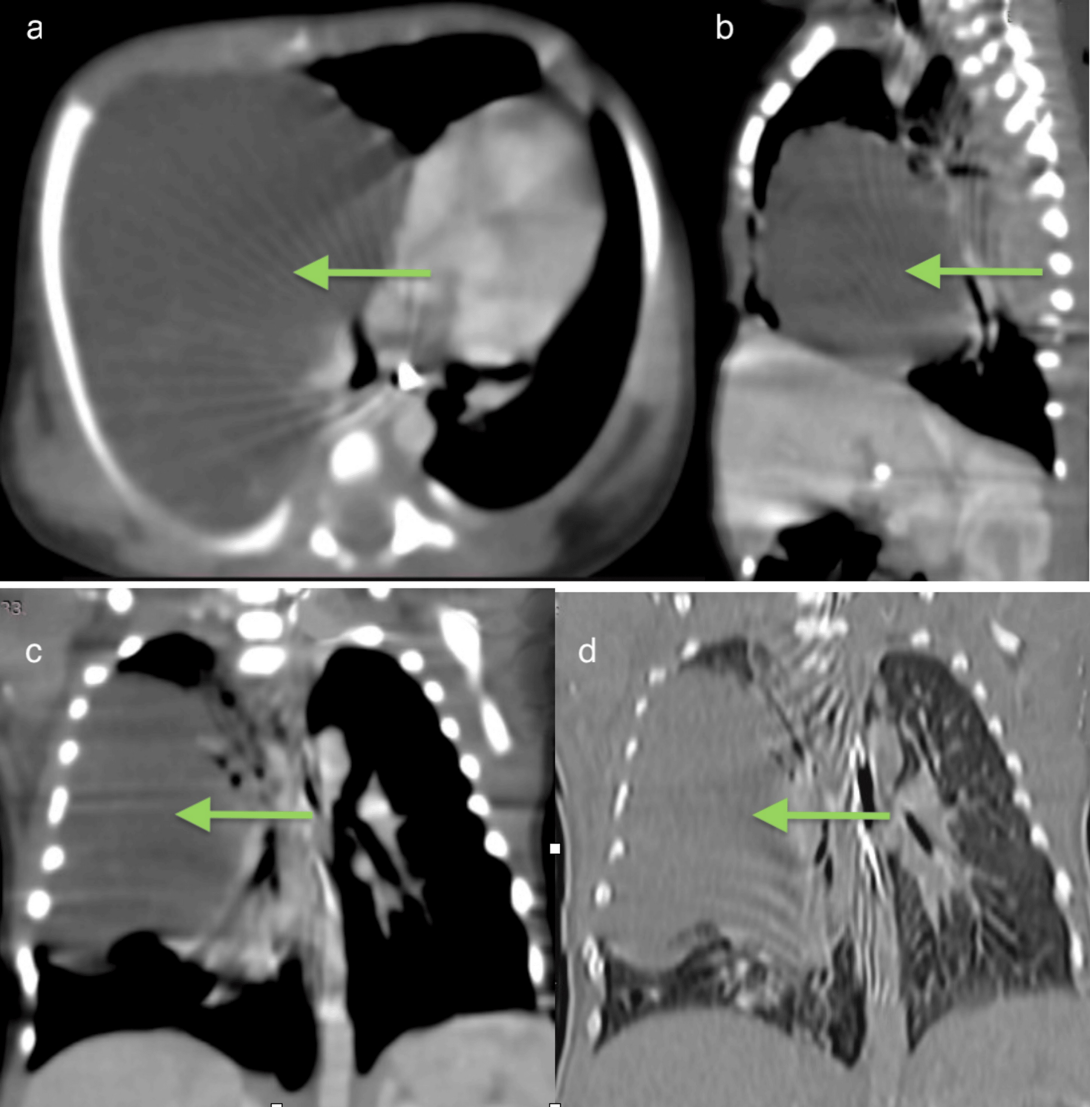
Multiplanar foetal contrast-enhanced CT images (axial, sagittal, and coronal views). (a) Axial image demonstrates a well-defined, large, multiloculated cystic lesion (arrow) involving the right middle lobe, causing splaying of the right upper and lower lobe bronchi with associated tracheomediastinal shift toward the left. Adjacent lung parenchyma shows collapse-consolidative changes. (b) Sagittal image demonstrates the craniocaudal extent of a multiloculated cystic lesion (arrow) involving the right middle lobe, with collapse-consolidation of adjacent lung. (c, d) Coronal reformatted images show a large multiloculated cystic lesion (arrow) in the right middle lobe, with separation of the right upper and lower lobe bronchi and resultant leftward tracheomediastinal displacement. Surrounding lung parenchyma demonstrates compressive collapse and consolidation.
